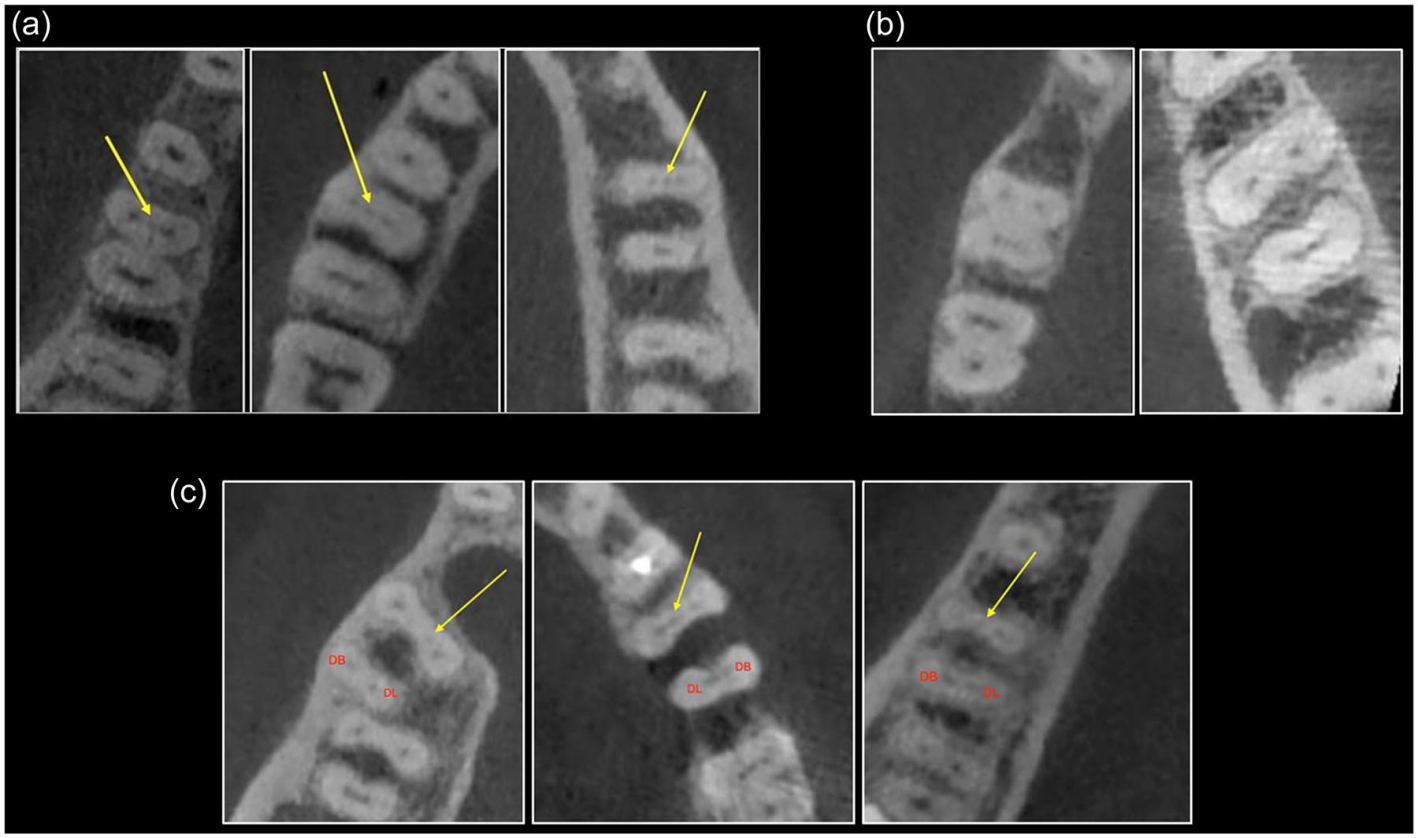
(a) Axial view of the CBCT images with middle mesial canal, (b) axial view of the CBCT images without middle mesial canal, and (c) axial view of the CBCT images with middle mesial canal and an additional distal canal. The yellow arrows point to the middle mesial canal. DB, distobuccal; DL, distolingual.
